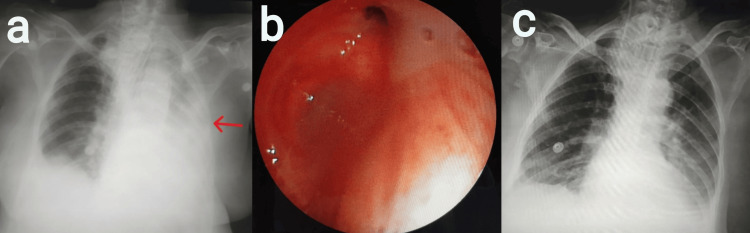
a) Chest radiograph revealed a left-sided white-out lung; b) bronchoscopy revealed mucus plugs blocking the left mainstem bronchus, left upper lobe (LUL) bronchus, and left lower lobe (LLL) bronchus; and c) post bronchial wash, a chest radiograph was performed, which showed the complete expansion of the left lung
